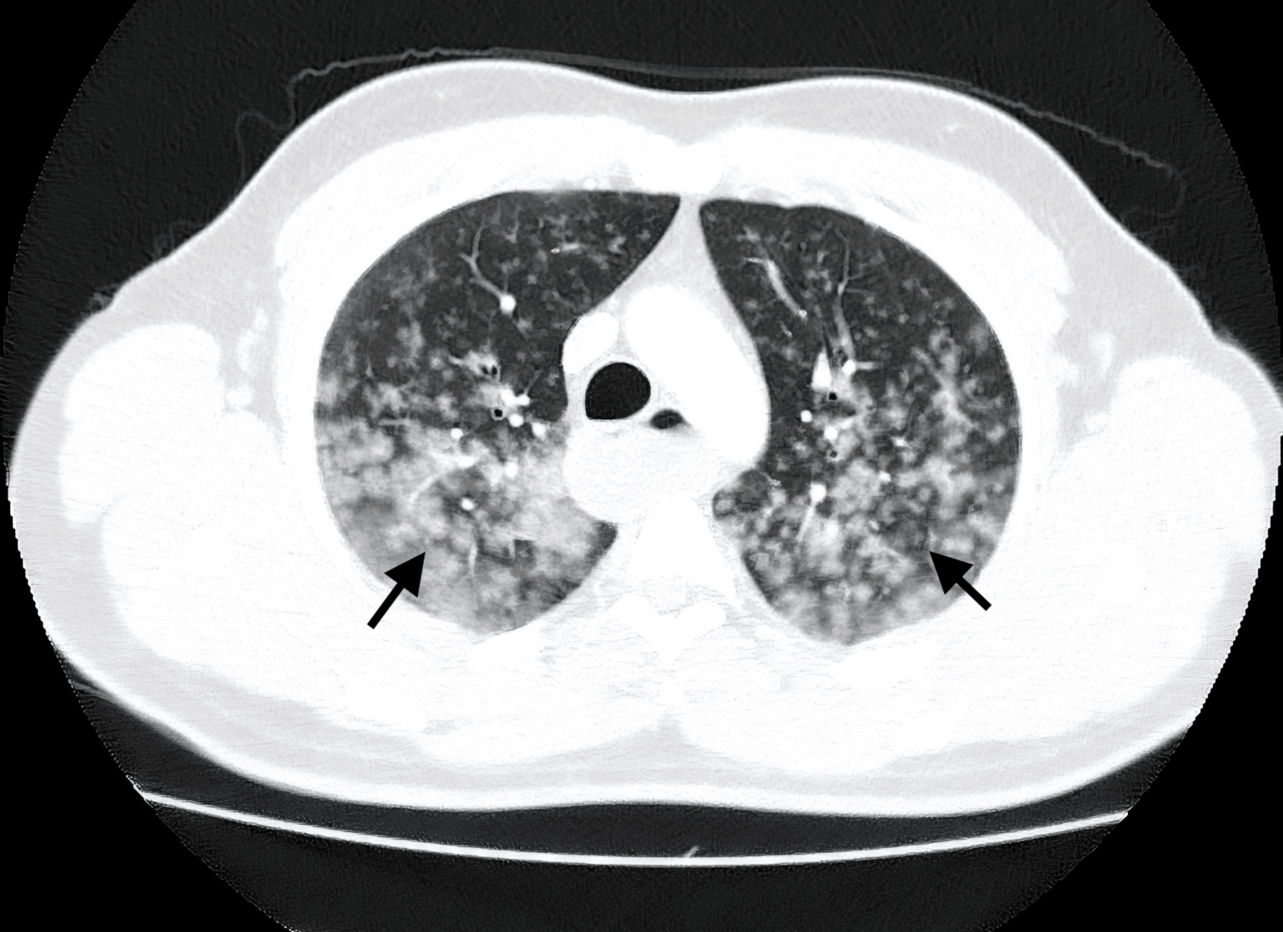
Axial non-enhanced CT image in lung window at the level of the aortic arch. There are bilateral ground glass opacities in a centrilobular and perivascular distribution in the posterior aspect of both lungs (arrows). These findings are compatible with diffuse pulmonary hemorrhage.
